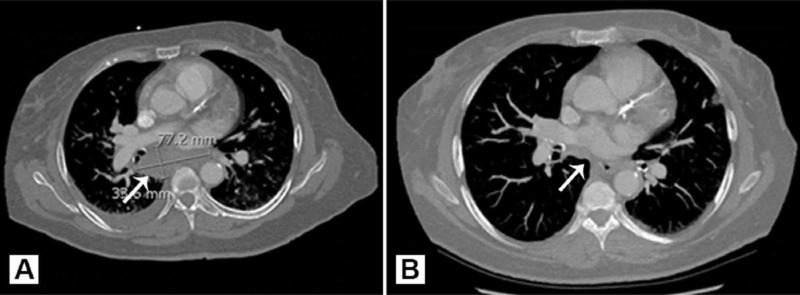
CT imaging of sub-carinal medialstinal mass (arrows) at (A) presentation and (B) following five months of therapy
